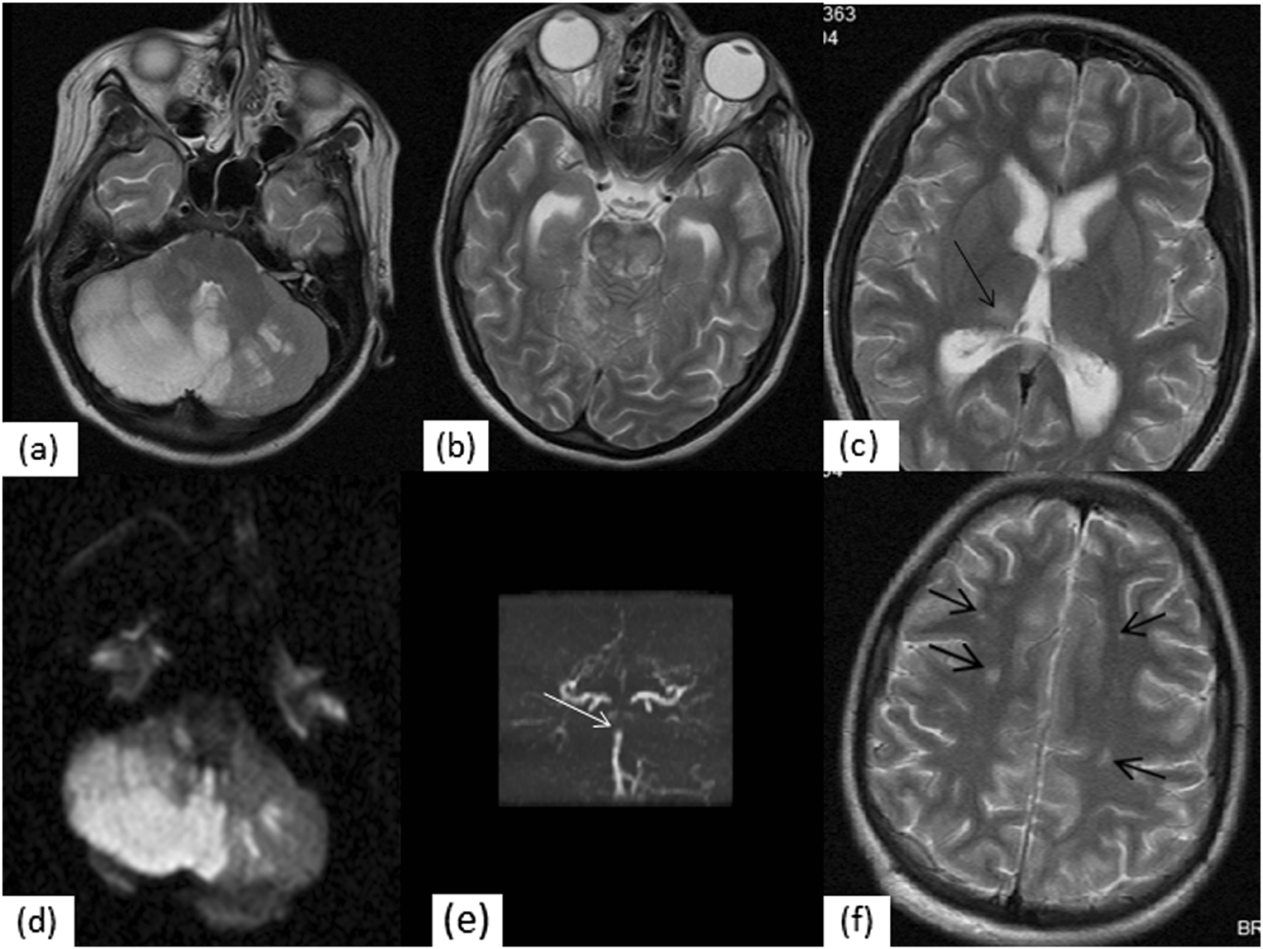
Infarction in a 14 year old with NPSLE of the a cerebellum, b brainstem, and c right posterior thalamus (*arrow*) diagnosed on the T2-weighted MR images. Cerebellar swelling compressing the fourth ventricle resulted in mild hydrocephalus. d The diffusion-weighted imaging revealed a high signal lesion in keeping with an acute infarct. e A signal void of the distal basilar artery (*white arrow*) on the MRA of the posterior circulation revealed a large vessel occlusion. f Multiple borderzone infarcts are seen within the deep white matter of the frontal lobes, some of which were acute. The anterior circulation was normal (not shown)
